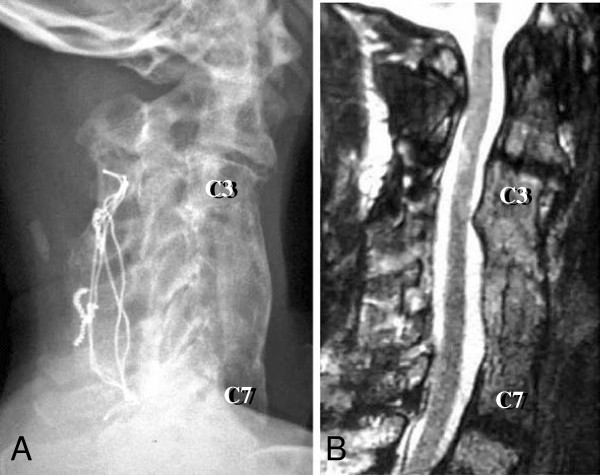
Two images taken six years after the fourth surgery. (A) A lateral view cervical radiogram showing successful interbody fusion. (B) A T2-weighted midsagittal magnetic resonance image showing mild disc degeneration at the adjacent C2-C3 and C7-T1 levels, but no spinal cord compression.
